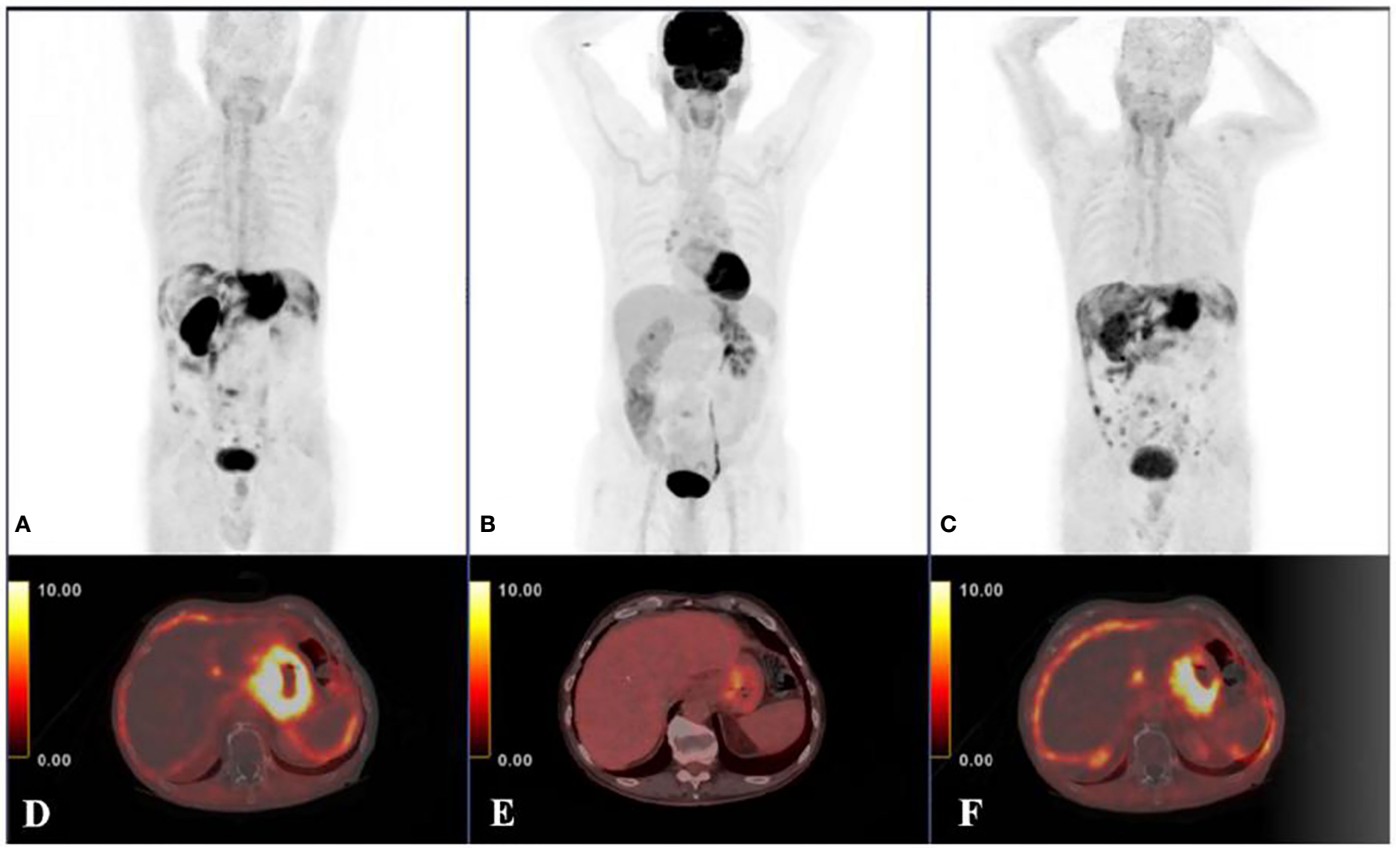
Another 78-year-old male diagnosed with poorly differentiated gastric adenocarcinoma, ^68^Ga-FAPI-04 PET/CT (A, D) and ^18^F-FDG PET/CT scans (B, E) revealed highly uptake of FAPI in the primary tumor and peritoneal carcinomatosis compared with FDG (primary tumor SUVmax 23 and 6.8; primary tumor tumor-to-background ratio;11.5 and 3.8; peritoneal-carcinomatosis SUVmax 7.5 and 2.3, respectively). The ^68^Ga-FAPI-04 PET/CT examination after 4 months of chemotherapy (C, F) showed the disease progression of this patient. The results demonstrated that ^68^Ga-FAPI-04 PET/CT was superior to ^18^F-FDG PET/CT in detecting primary gastric adenocarcinoma and peritoneal carcinomatosis with a gastric cancer origin. With permission from Kuten et al. (49).
